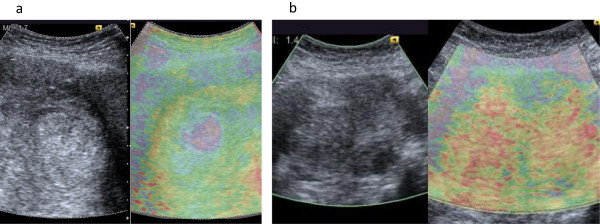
Ultrasound images of uterine leiomyosarcoma (a) and leiomyoma (b). Left: US B-mode image Right: VTI. The results of gray-scale and VTI are shown in Figure
